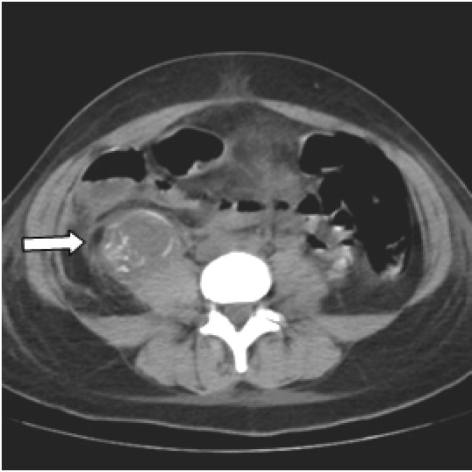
Unenhanced lower abdomen CT scan shows a retroperitoneal hypodense lesion with internal hyperdense bony elements, in contact with the right psoas muscle (arrow).
